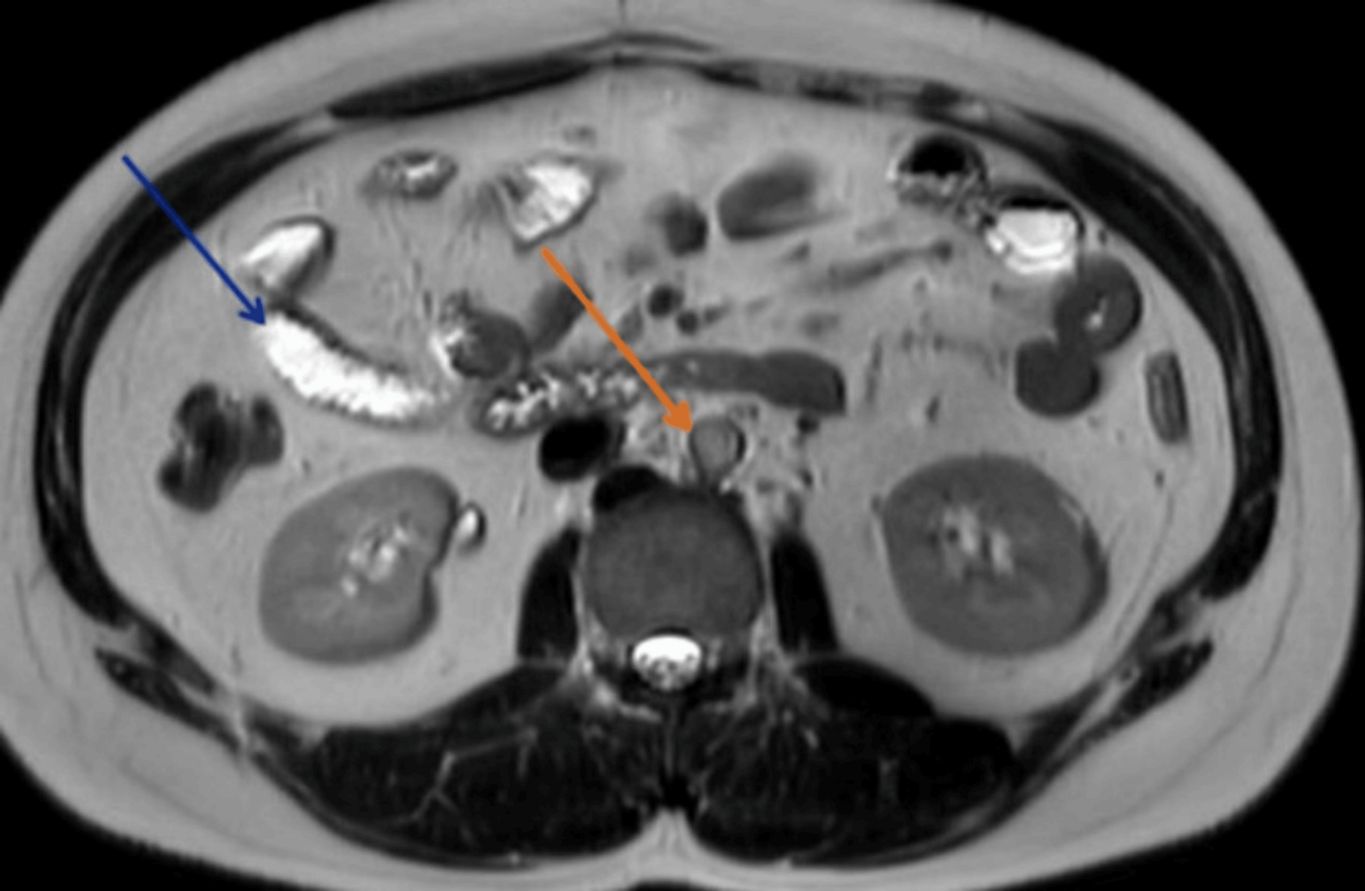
Magnetic resonance cholangiopancreatography An axial T2-weighted MR image shows a conglomerate of necrotic lymph nodes (orange arrow) in the portacaval region, just posterior to the pancreatic head. This nodal mass is causing extrinsic compression and smooth tapering of the distal common bile duct (blue arrow), with resultant upstream biliary dilatation. This appearance is classic for benign extrinsic compression, in this case from tuberculous lymphadenitis.
